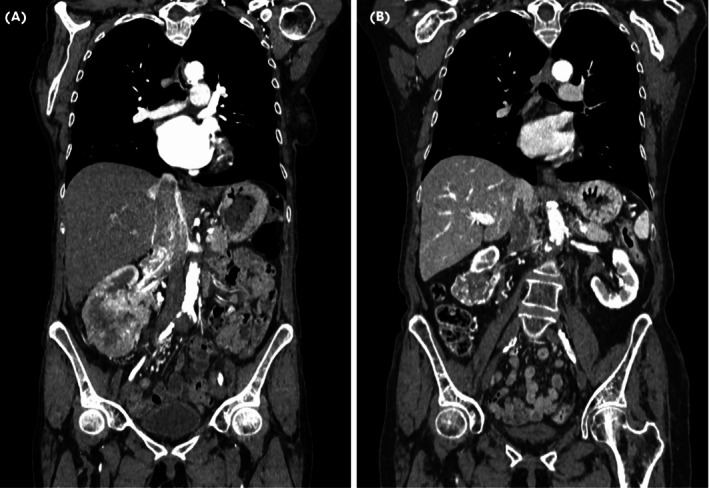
Coronal CT reconstructions at (A) baseline showing large right renal mass and supra‐diaphragmatic extension of tumour thrombus in the IVC (Mayo Level IV) and (B) at 15 months showing shrinkage of renal mass and tumour thrombus to below level of hepatic veins (Mayo Level II).
